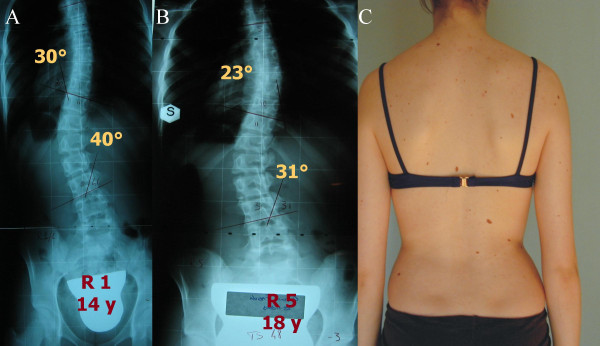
Typical result in this study. A. Starting x rays. B. Final x-rays. C. Aesthetic final result. F decreased from 30-40° at 14 years of age, Risser 1, pre-menarchal to 23-31° at 18 years, Risser 5.
